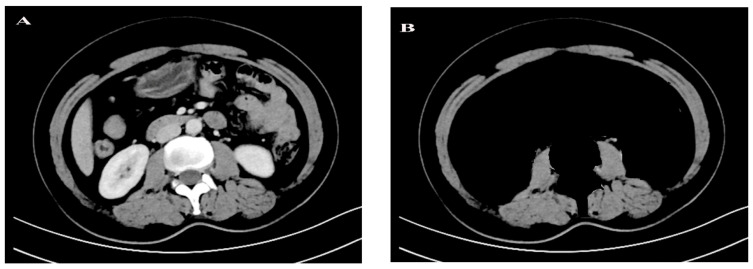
An example of abdominal computed tomography (CT) image. (A) The original CT image. (B) Skeletal muscle are quantified range of (−29 to + 150 Hounsfield units), and they were outlined along the epimysium that surrounded the muscles.
